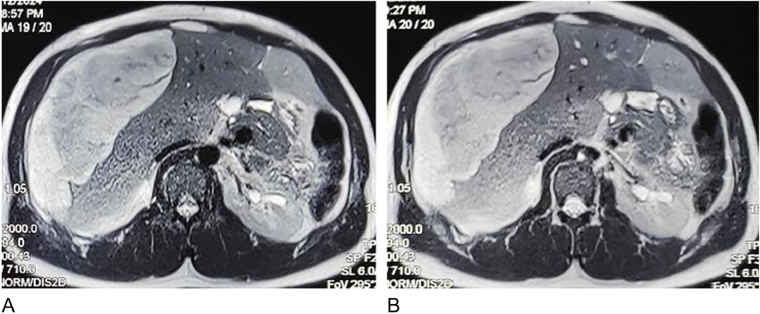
A & B: Magnetic resonance imaging (MRI) of the Abdomen and Pelvis (T2-weighted) performed one week after admission (15/09/2024), shows a well-contained subcapsular hepatic hematoma without interval expansion, consistent with stabilization under conservative management.
